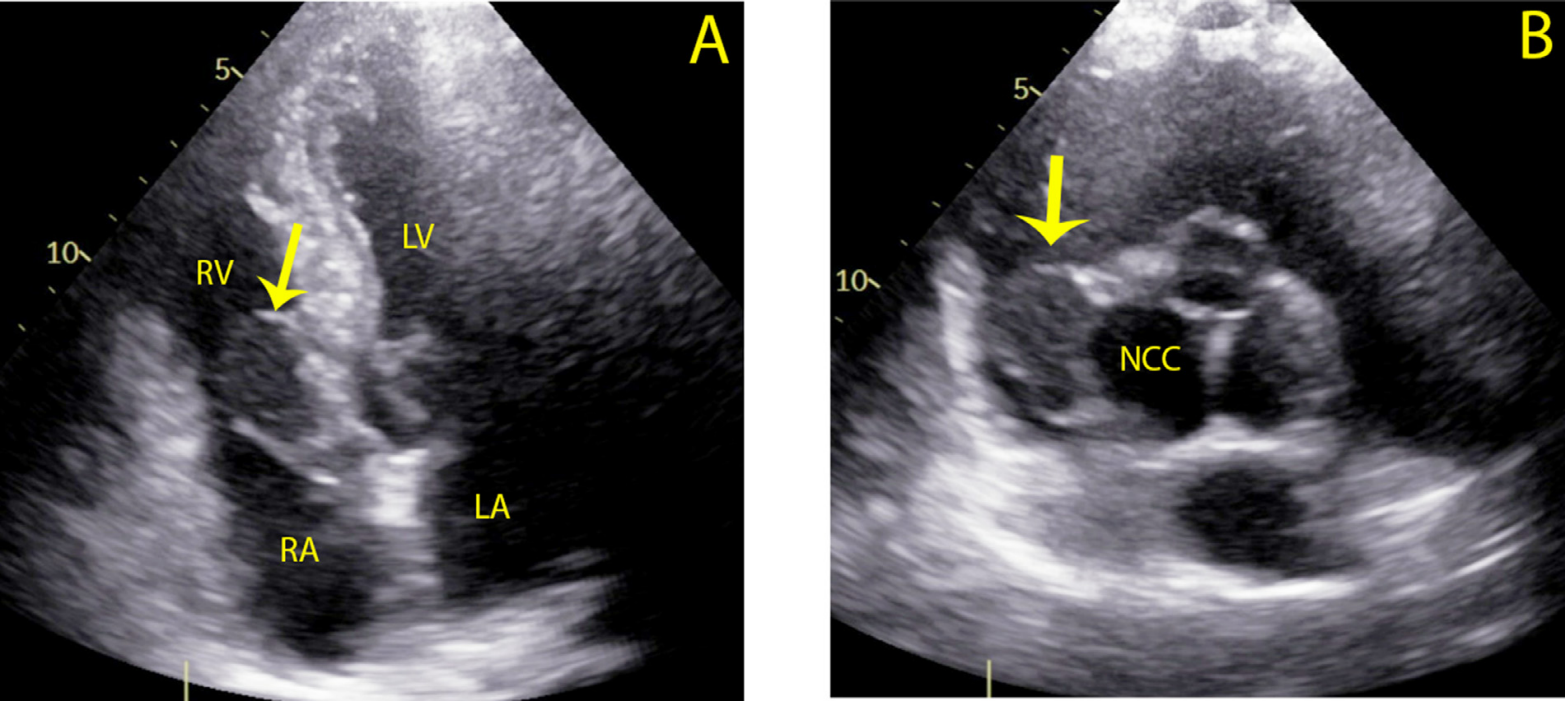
Transthoracic Echocardiography with image of hypo-echogenic structure (yellow arrow) in the right ventricular (RV) cavity in apical four-chamber view (panel A) and parasternal short-axis view (panel B). *LV: left ventricle, LA: left atrium, NCC: non-coronary cusp, RV: right ventricle, RA: right atrium.*
